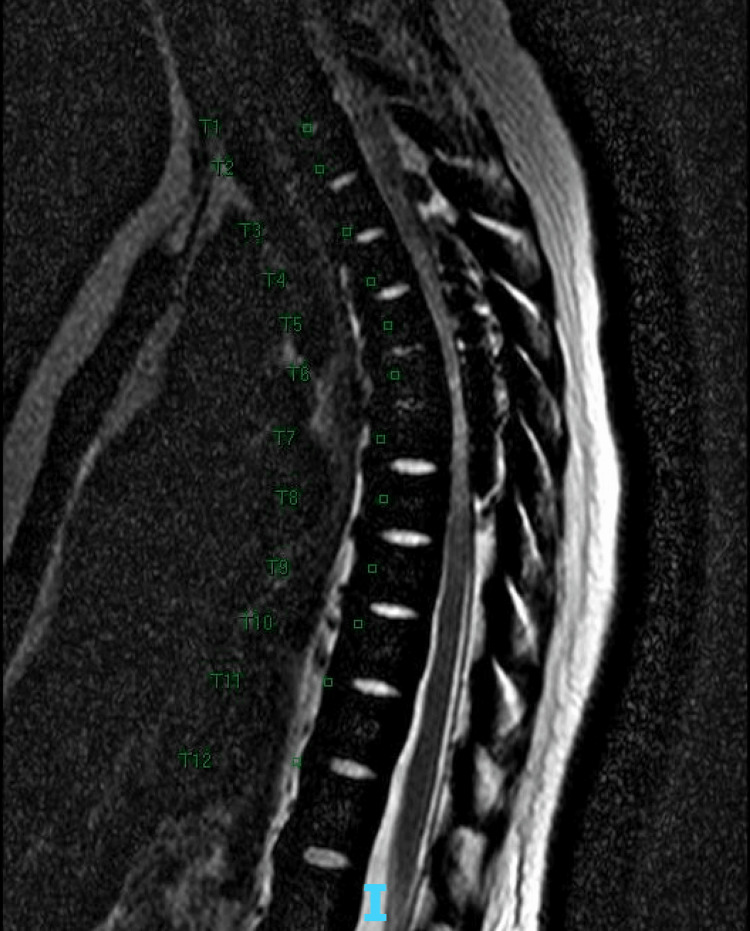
Initial thoracolumbar MRI showing severe cord compression from T3-T8 vertebrae.
